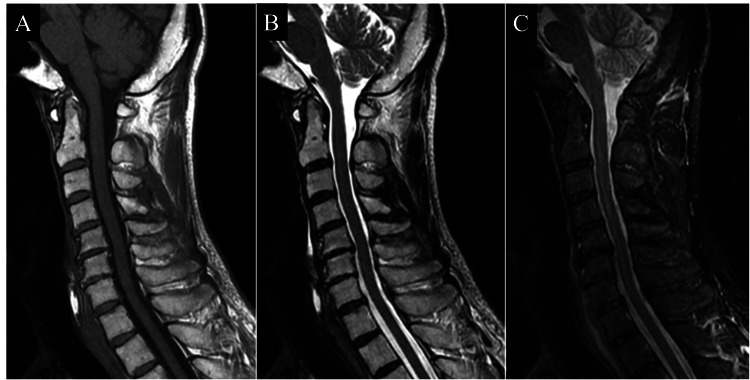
Cervical spine MRI at three years post-injury. A: Sagittal T1-weighted image. B: Sagittal T2-weighted image. C: Sagittal short tau inversion recovery (STIR) image. In A–C, the signal changes associated with traumatic changes at the time of injury observed have resolved.
